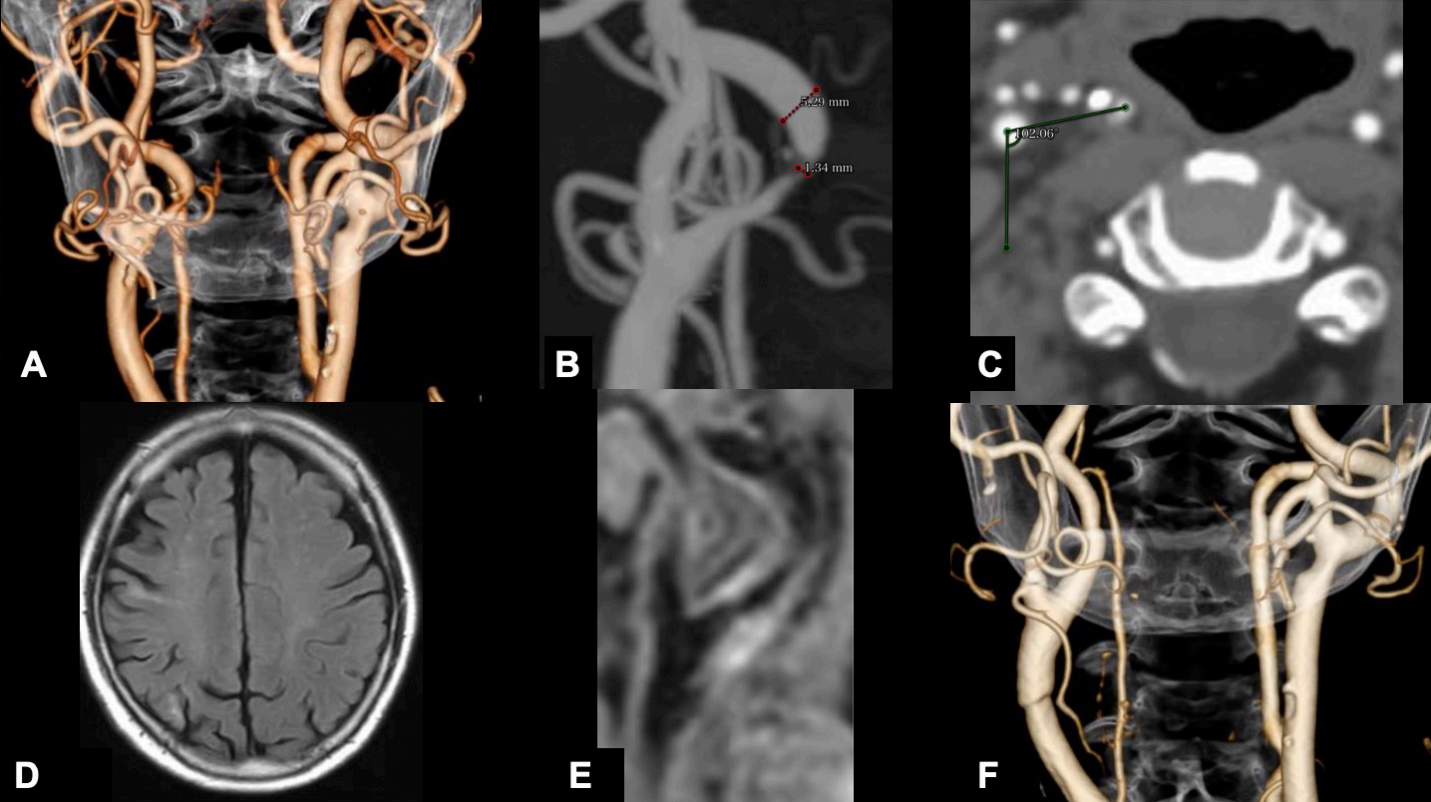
(A) Anteroposterior three-dimensional computed tomography angiography (3D-CTA) showed the internal carotid artery (ICA) medially to the external carotid artery (ECA). (B) CTA showed 74% ICA stenosis according to North American Symptomatic Carotid Endarterectomy Trial criteria. (C) The angle of twisting was measured in the axial view on CTA by computing the degree of medial deviation from the line connecting the centers of the ICA and ECA to the line parallel to the sagittal plane at the center of the ECA. The preoperative twist angle was 102°. (D) Fluid-attenuated inversion recovery imaging showed a chronic cerebral infarction in the right parietal lobe. (E) Black-blood T1-weighted image showed a high-intensity signal, indicating an unstable vulnerable plaque. (F) Postoperative anteroposterior 3D-CTA showed improvement of the ICA stenosis without repositioning.
